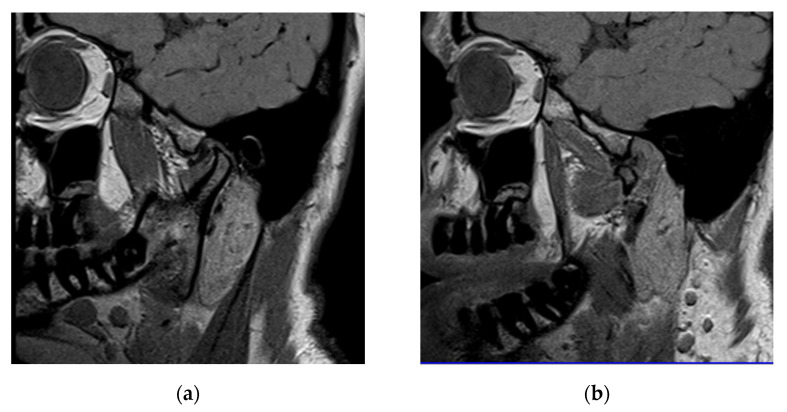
Sagittal (a) closed and (b) open mouth proton density MRI of the left joint: anterior disk displacement with reduction (DDR), condylar bone changes (flattened condyle, with posterolateral compression of the left condylar head, lateral resorption of the left condylar head with posterior position of the condyle in the articular fossa) and thickened posterior band disk shape.
